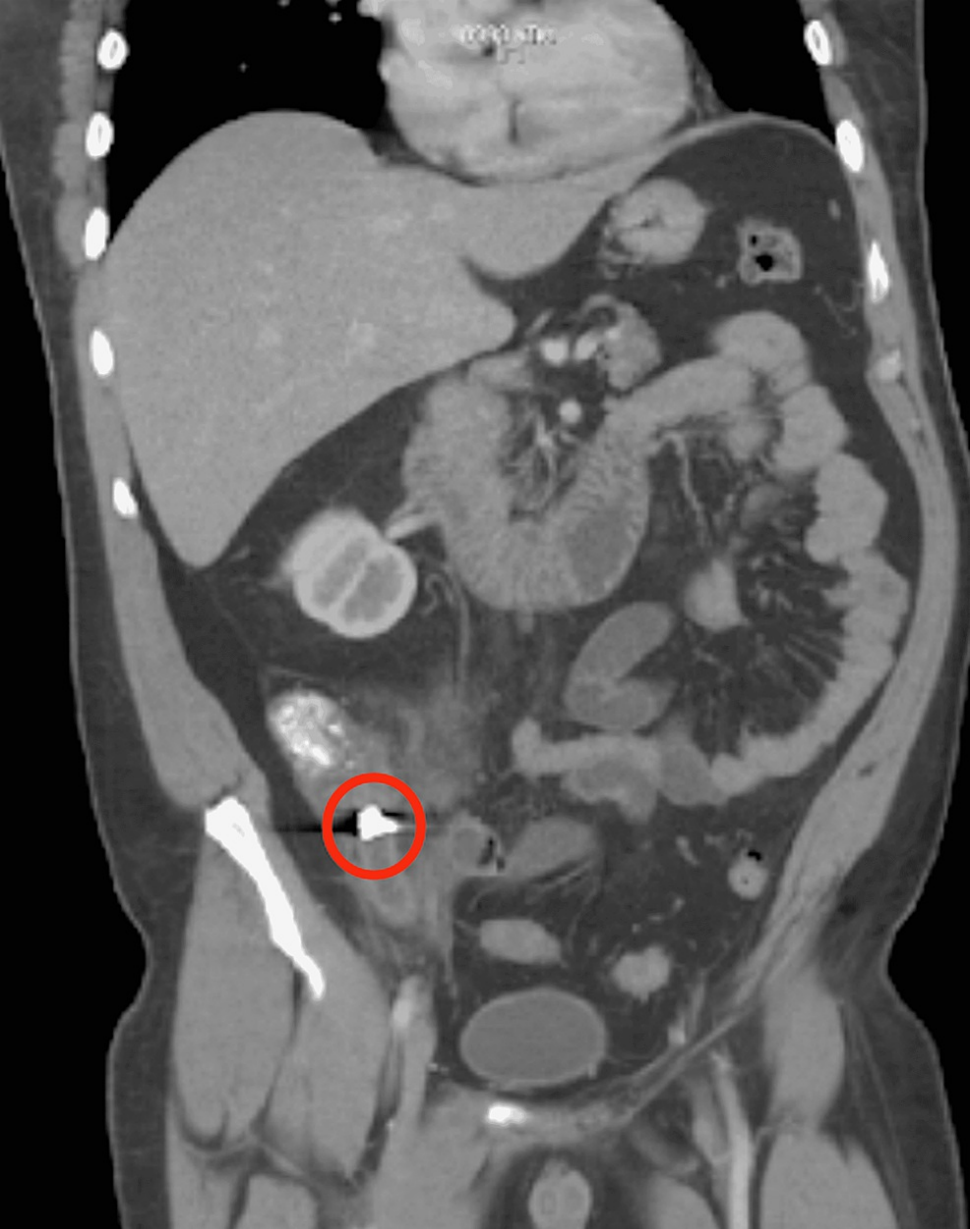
Computed tomography scan showing metallic object in appendix
